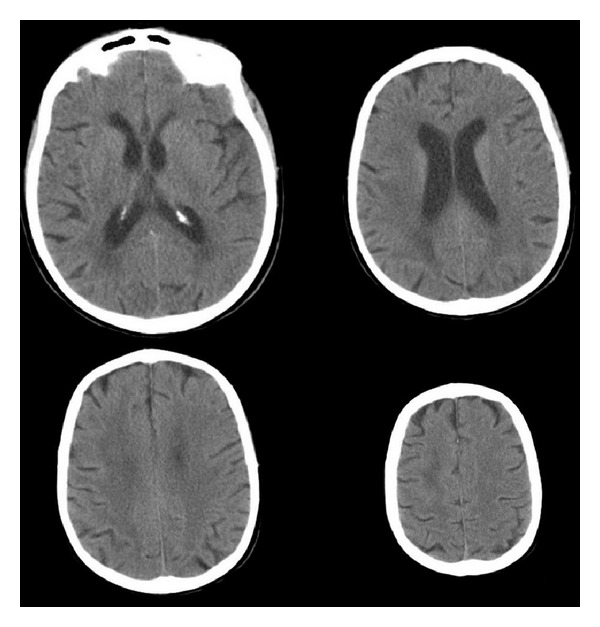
CT scan showed no sign of hemorrhage.
